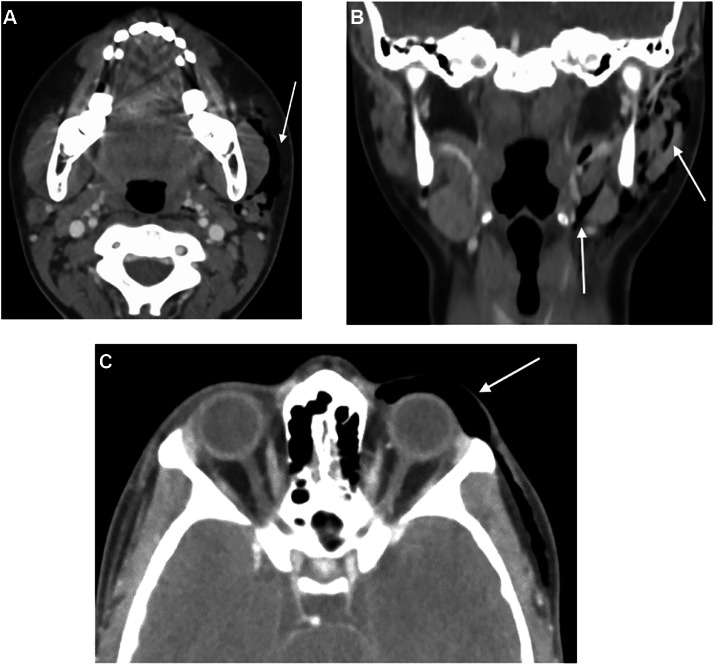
Aaxial and coronal CT showing left pneumoparotid, palpebral and cervical emphysema.
